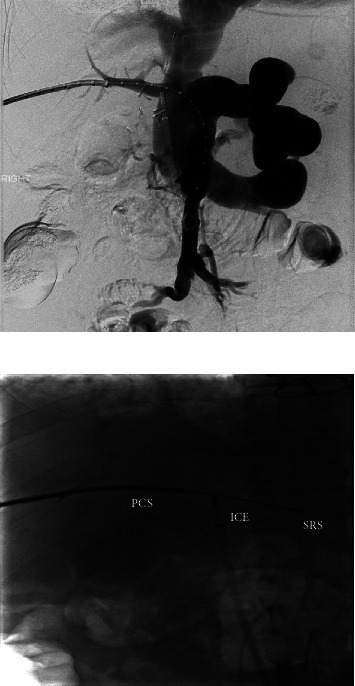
a & b: portal vein access. Challenging and relatively central access is due to diminutive portal vein branches. Intravascular ultrasound guidance.
